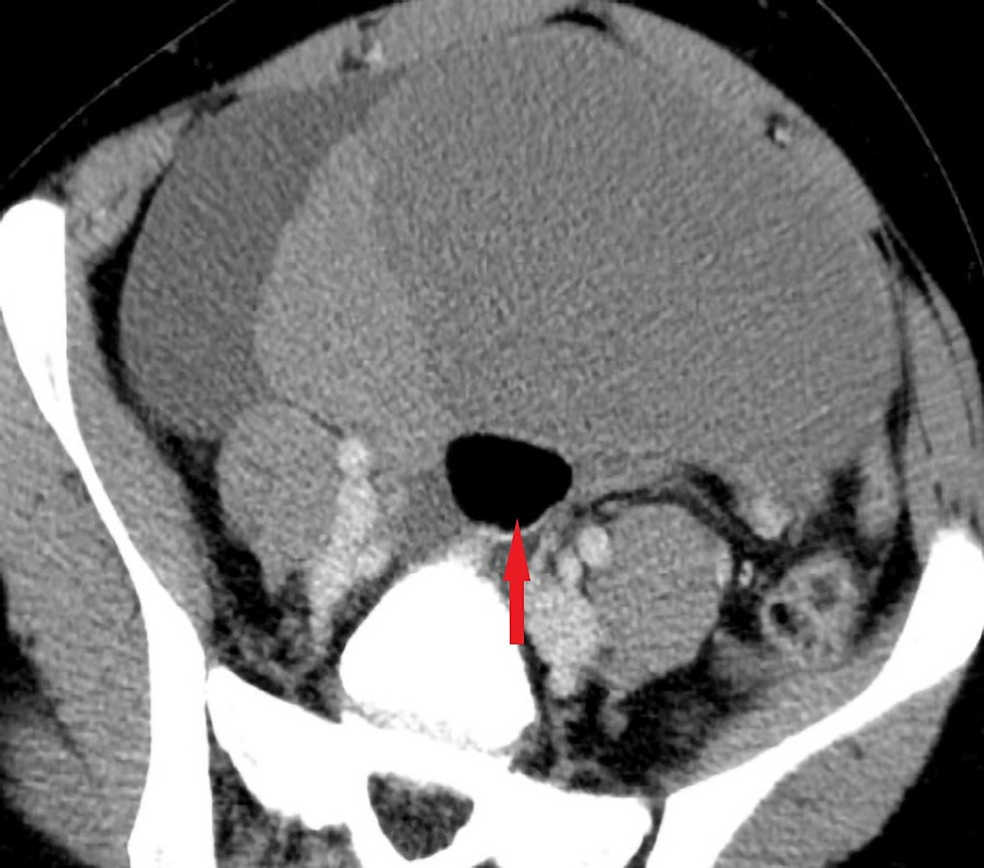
Axial post-contrast CT demonstrates a complex left ovarian mass with solid and cystic components, notice a globule of fat (red arrow).
